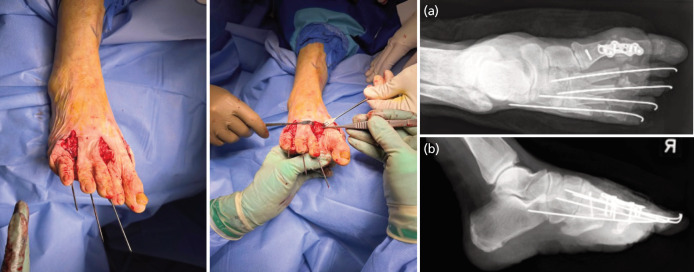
Intra-operative photographs of the right forefoot reconstruction, as well as post-operative, (a) AP and (b) lateral radiographs.
